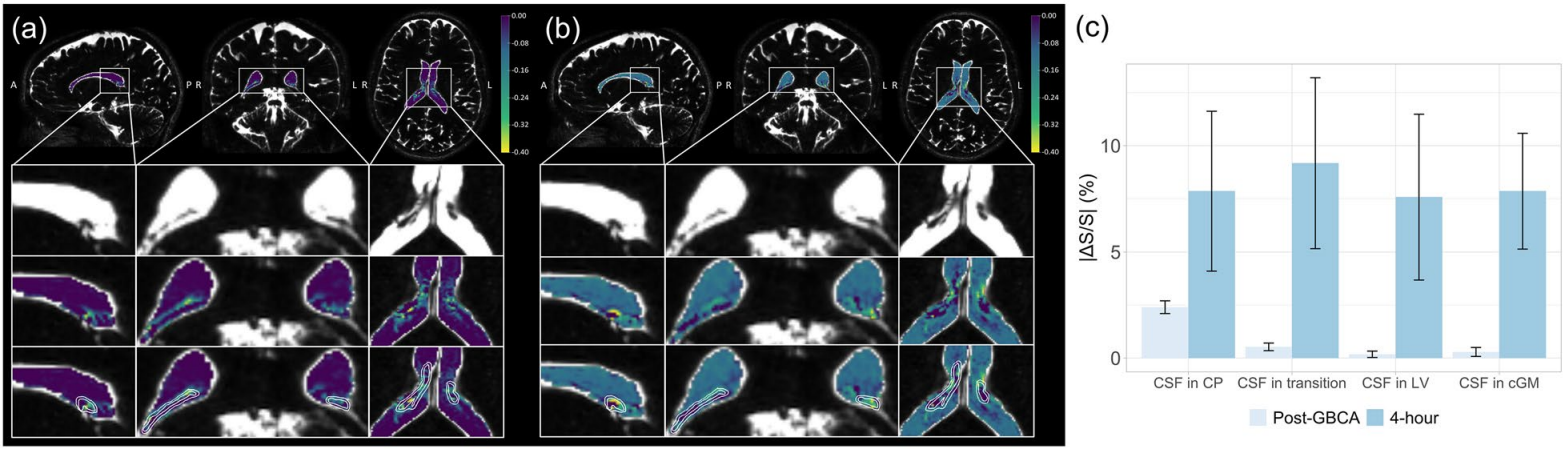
Dynamic signal changes in the CSF around the choroid plexus (CP) measured by cDSC MRI. a Representative maps of relative signal changes (ΔS/S) averaged over 50–250 s after intravenous GBCA administration in the lateral ventricle (including the CP) from a healthy human subject (33 years old, female) are shown. Results were overlaid on the pre-GBCA cDSC MRI images. The regions in the white boxes on the first row are magnified on the second to fourth rows. The second row shows the original pre-GBCA cDSC MRI images. The third row displays cDSC MRI images overlaid with ΔS/S in the lateral ventricle. On the fourth row images, the CP and transition area between CP and CSF were outlined with white contours. A: anterior; P: posterior; R: right; L: left. The scale bar indicates the range of ΔS/S. Note that the cDSC MRI method has a negative contrast for GBCA-induced signal changes in the CSF. b Representative maps of ΔS/S approximately 4 h after intravenous GBCA administration in the lateral ventricle (including the CP) from the same healthy human subject are shown. Results were overlaid on the pre-GBCA cDSC MRI images. c Average absolute ΔS/S in the CSF immediately and 4 h after intravenous GBCA administration measured by cDSC MRI from all healthy human subjects (n = 25). The CSF signal changes in four ROIs were calculated: the choroid plexus (CP), transition area between CP and CSF (transition), the rest of lateral ventricle (LV), and the perivascular space of occipital cortical grey matter (cGM). The error bars represent inter-subject standard errors. Quantitative results are reported in Tables 2 and 3. Note that absolute ΔS/S is displayed here for easier comparison with subsequent results. cDSC: dynamic-susceptibility-contrast-in-the-CSF; GBCA: gadolinium-based-contrast-agent; CSF: cerebrospinal fluid
